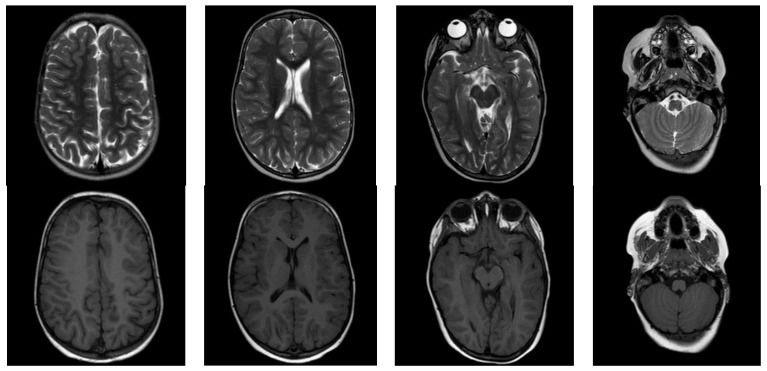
Serial MRIs (axial T2- and T1-weighted images) of the individual at 3 years of age show normal myelination. No abnormalities are depicted here.
